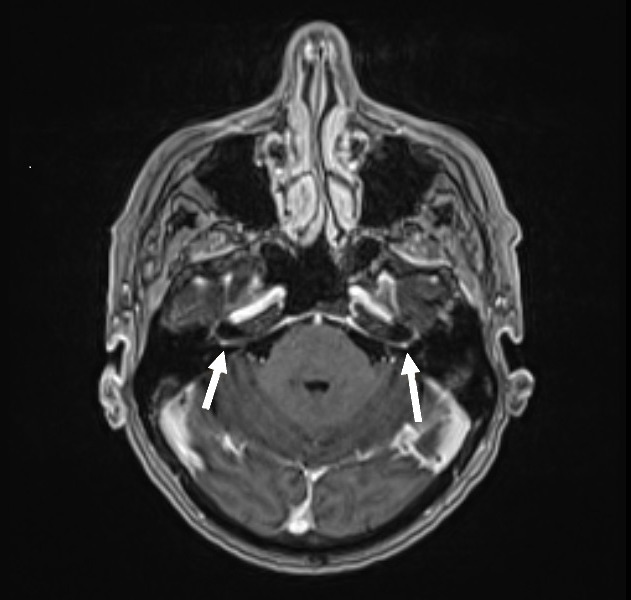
Magnetic resonance imaging (1.5T) findings: an axial contrast-enhanced thin section (after i.v. gadolinium application). The T1-weighted image shows bilateral intrameatal facial nerve enhancement (arrows).
